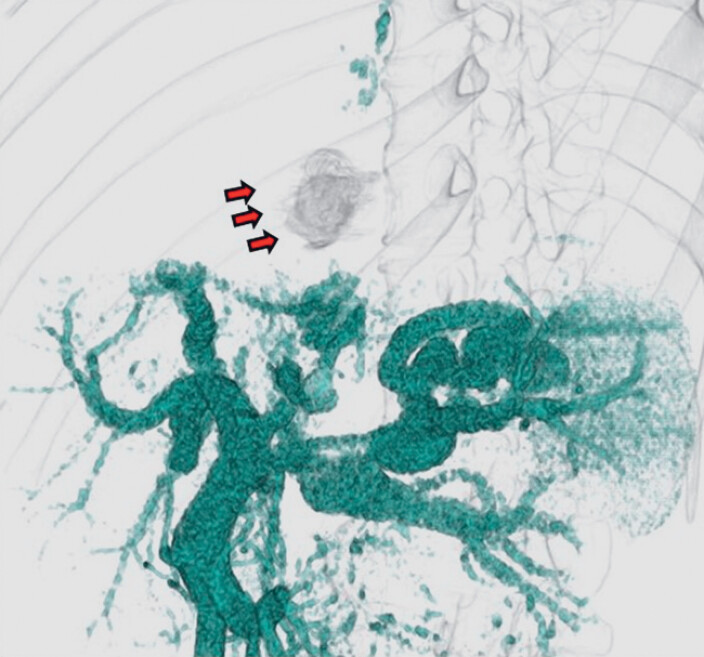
3D computed tomography image 1 week after the procedure showing the coils that had been placed (red arrows) and no further evidence of the varices.
